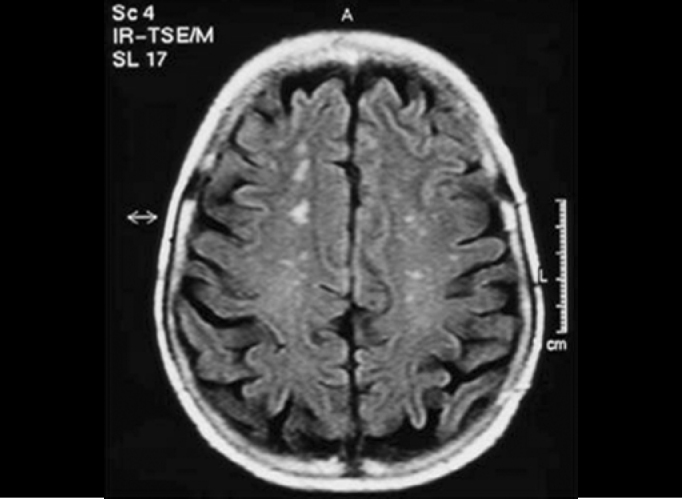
Hyperintense subcortical lesions - MR (FLAIR).
